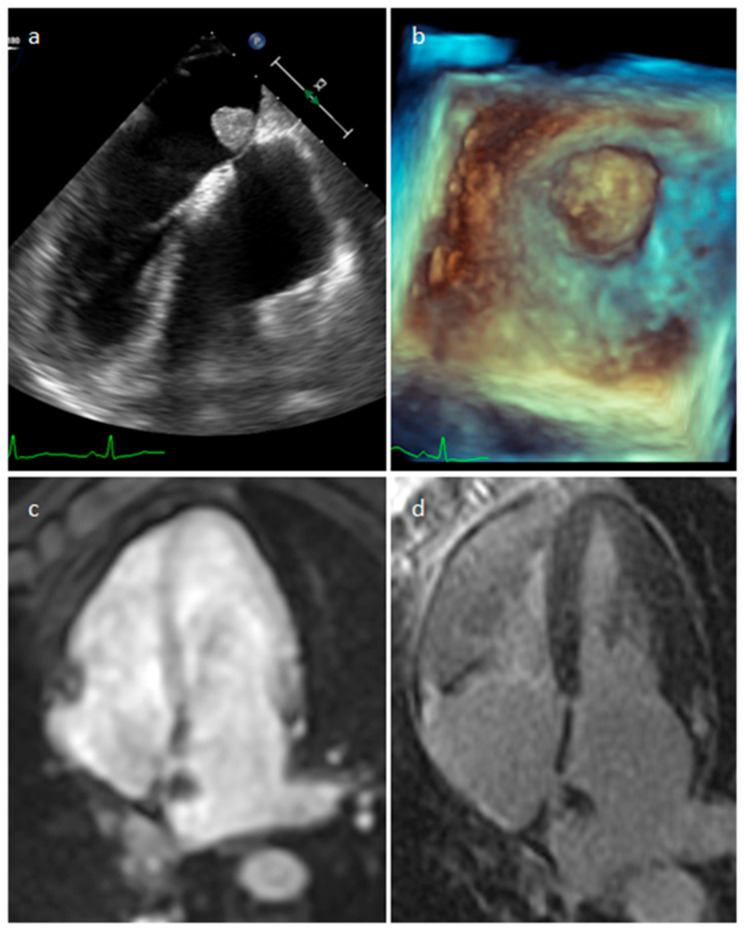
Left atrial myxoma at 2D (a) and 3D (b) transesophageal echocardiography, perfusion (c) and late gadolinium enhancement (d) MR imaging.
